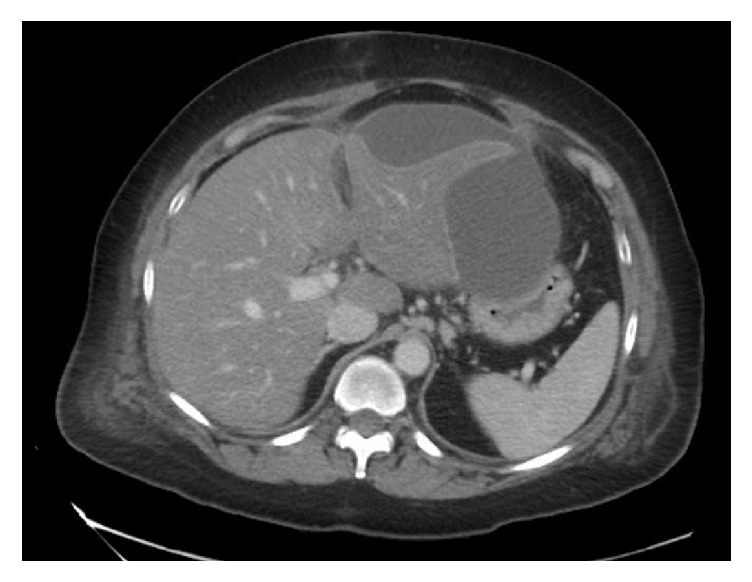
CT abdomen demonstrating subcapsular collection.
